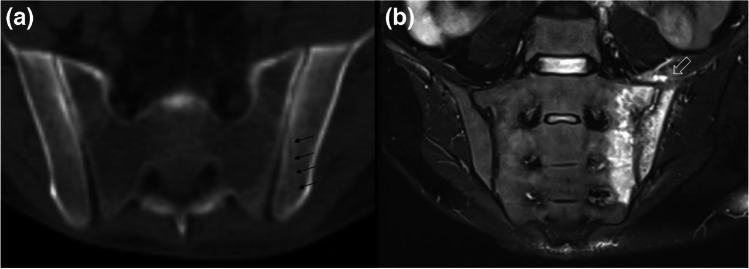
Axial CT image (a) depicting the needle track (black arrows) in a patient who underwent bone marrow biopsy and later developed postbiopsy septic sacroiliitis. Coronal STIR (b) image shows unilateral, extensive bone marrow edema on both sacral and iliac sides of the left sacroiliac joint, with joint effusion causing capsular distension (open arrow) and associated inflammatory signal changes in the left iliacus muscle. Pronounced periarticular inflammatory soft tissue changes, extensive edema, and asymmetric unilateral involvement are in favor of septic sacroiliitis and the needle track in the CT image acquired prior to start of clinical symptoms suggests iatrogenic seeding of infection in this patient. Joint fluid cultures revealed *S. aureus* as the etiologic microorganism
